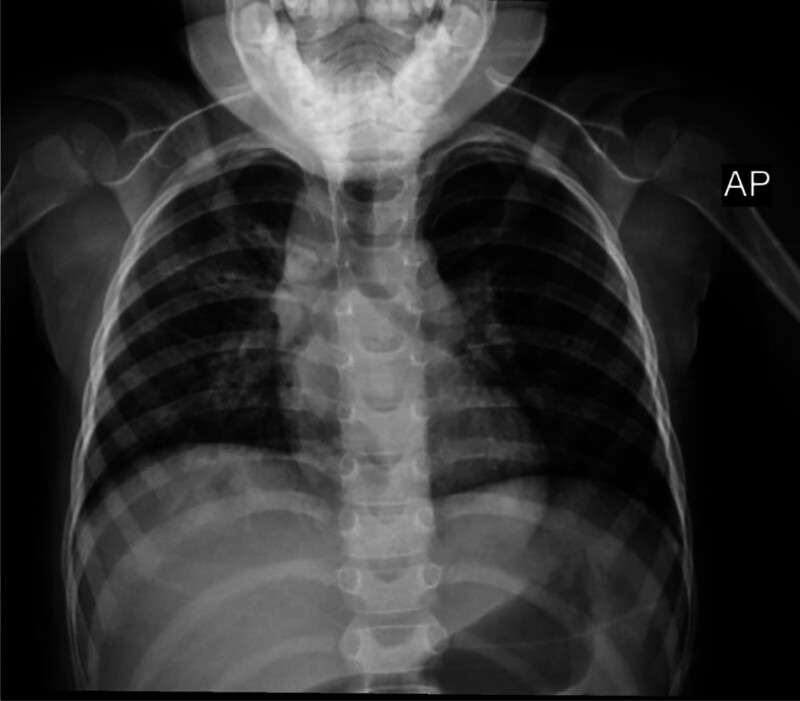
AP chest x-ray taken while the patient was in the supine position, showing right lower lobe opacities, particularly in the right retrocardiac region, suggesting airspace disease, mostly consistent with right lower lobe pneumonia. AP = anteroposterior.
